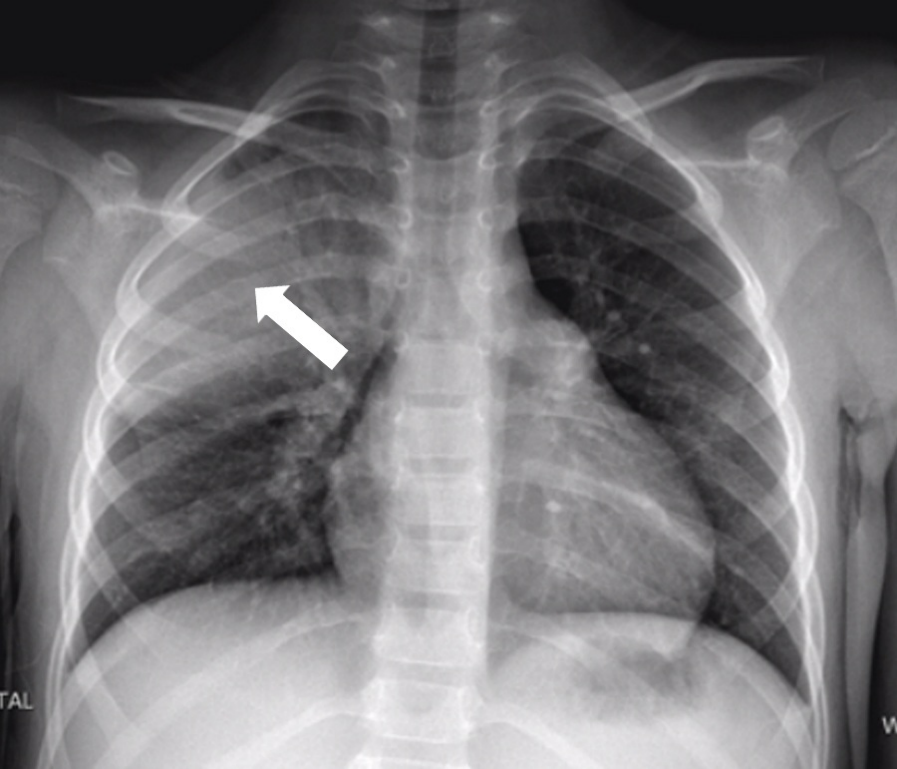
Chest X-ray – large focal area of consolidation seen in the right upper mid zone, consistent with right-upper lobe pneumonia.
